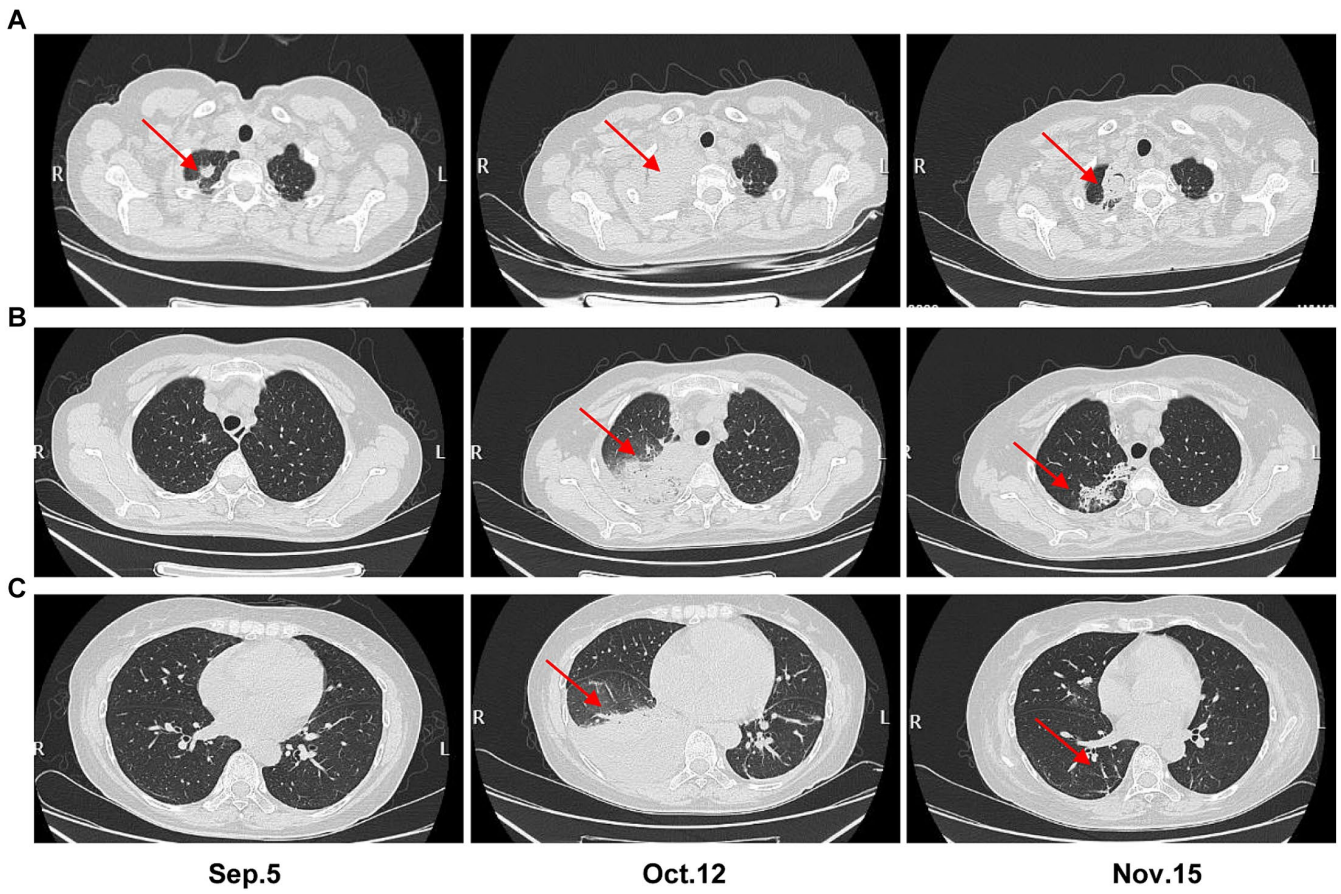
Chest CT scans at three time points (on admission, Oct.12, and Nov.15). (A–C) are the imaging findings of the apex of the right lung, the lower lobe of the right lung and the site of pleural effusion, respectively.
